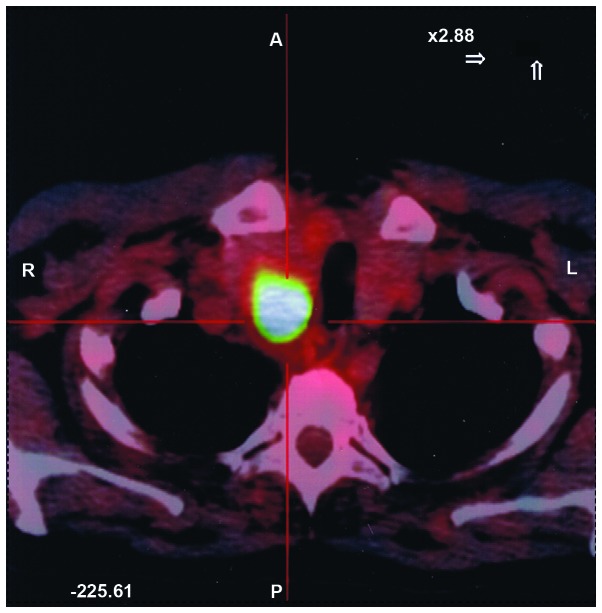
Positron emission tomography scan showing heterogeneous uptake in the right lobe of the thyroid gland (SUV 10.6).
